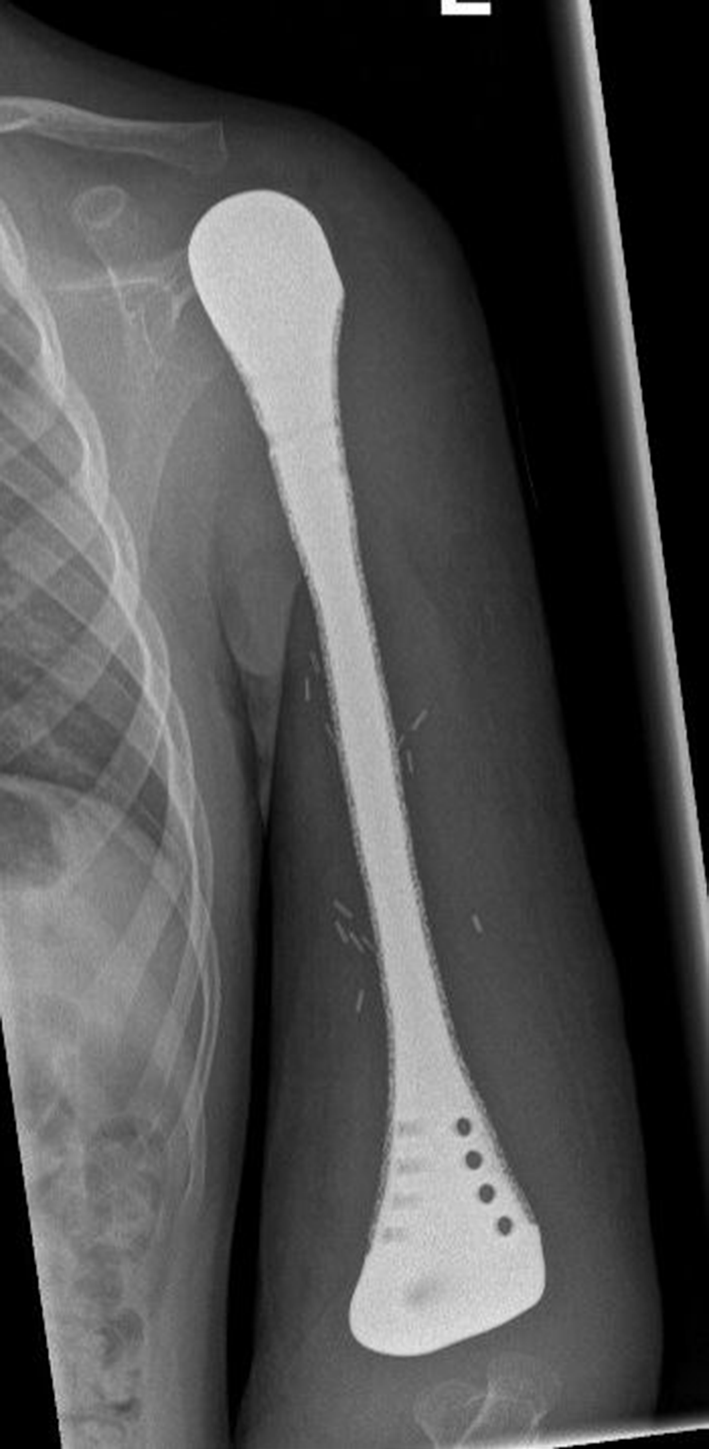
Postoperative radiograph of a total humerus reconstruction with an EPORE^®^ coating (patient #7)
